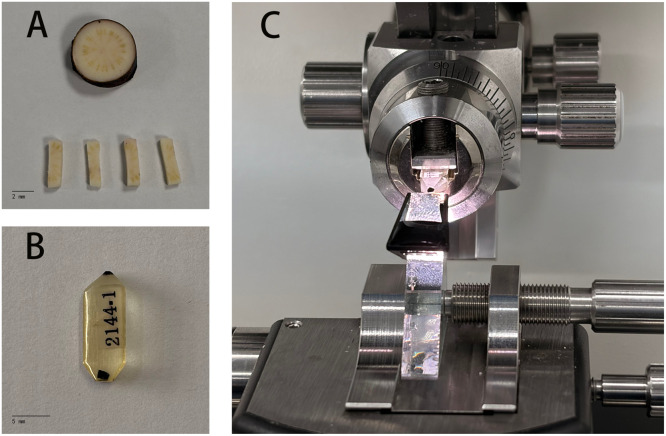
Resin embedding and sectioning procedure for soft X-ray imaging.
